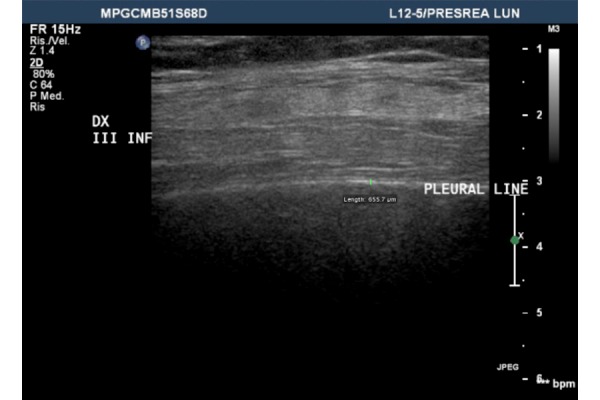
Lung Ultrasound (LUS): High frequency transducer (12 Mhz) linear probe positioned on anterior right axillary line (middle lobe). Normal hyperechoic pleural line and complete absence of horizontal reverberations (A-lines) and also absence of ring down artifacts (B-lines) and comet tails.
